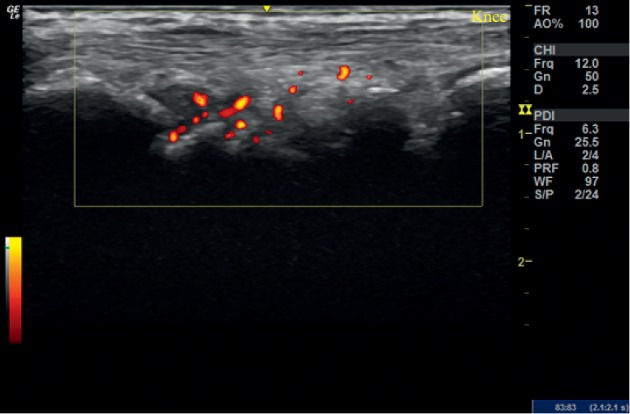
Initial ultrasound examination of the right wrist in case 1. Longitudinal view showing a power Doppler grade 2 synovitis. Multiple small carpal erosions were observed.
